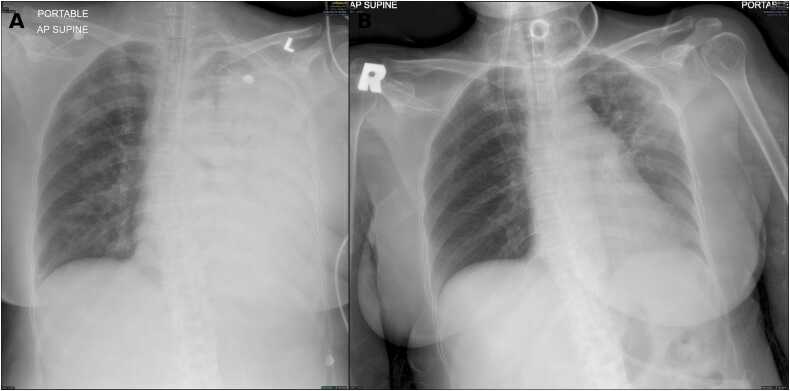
Portable anteroposterior chest radiographs obtained during hospitalisation. (a) Chest radiograph on Day 3 of admission demonstrating near-complete opacification of the left hemithorax. (b) Chest radiograph on Day 50 showing marked interval radiographic improvement with re-expansion of the left lung.
